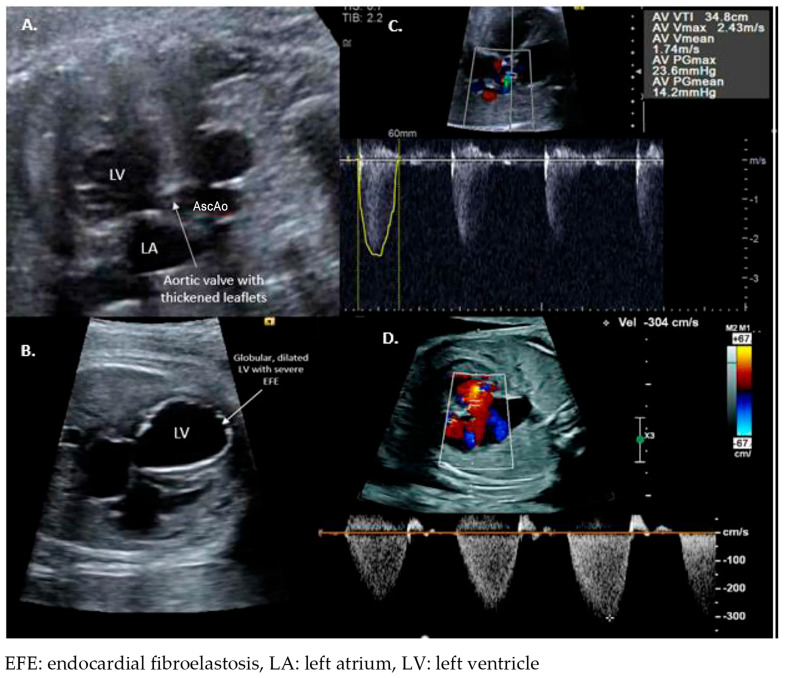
Fetus with critical aortic stenosis. Fetal echocardiogram performed at 29 wks GA. (A) Left ventricular outflow view demonstrating thickened aortic valve leaflets. Annulus measures mildly hypoplastic with a z-score of −2.4. (B) Four-chamber view demonstrating severely dilated, globular left ventricle with echobright endocardium consistent with endocardial fibroelastosis. (C) Doppler interrogation of the aortic outflow tract with a peak velocity of ~2.4 meters per second (m/sec) in the setting of severe left ventricular dysfunction. (D). Doppler interrogation of the mitral valve regurgitation predicting left ventricle systolic pressure of ~3 m/sec plus systemic venous pressure.
